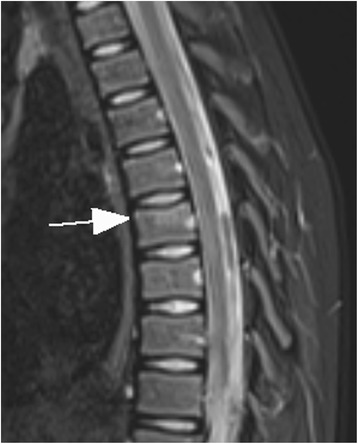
Close up sagittal STIR MR imaging shows oedema and slight impression of the upper endplate of T6 vertebral (*arrow*)
